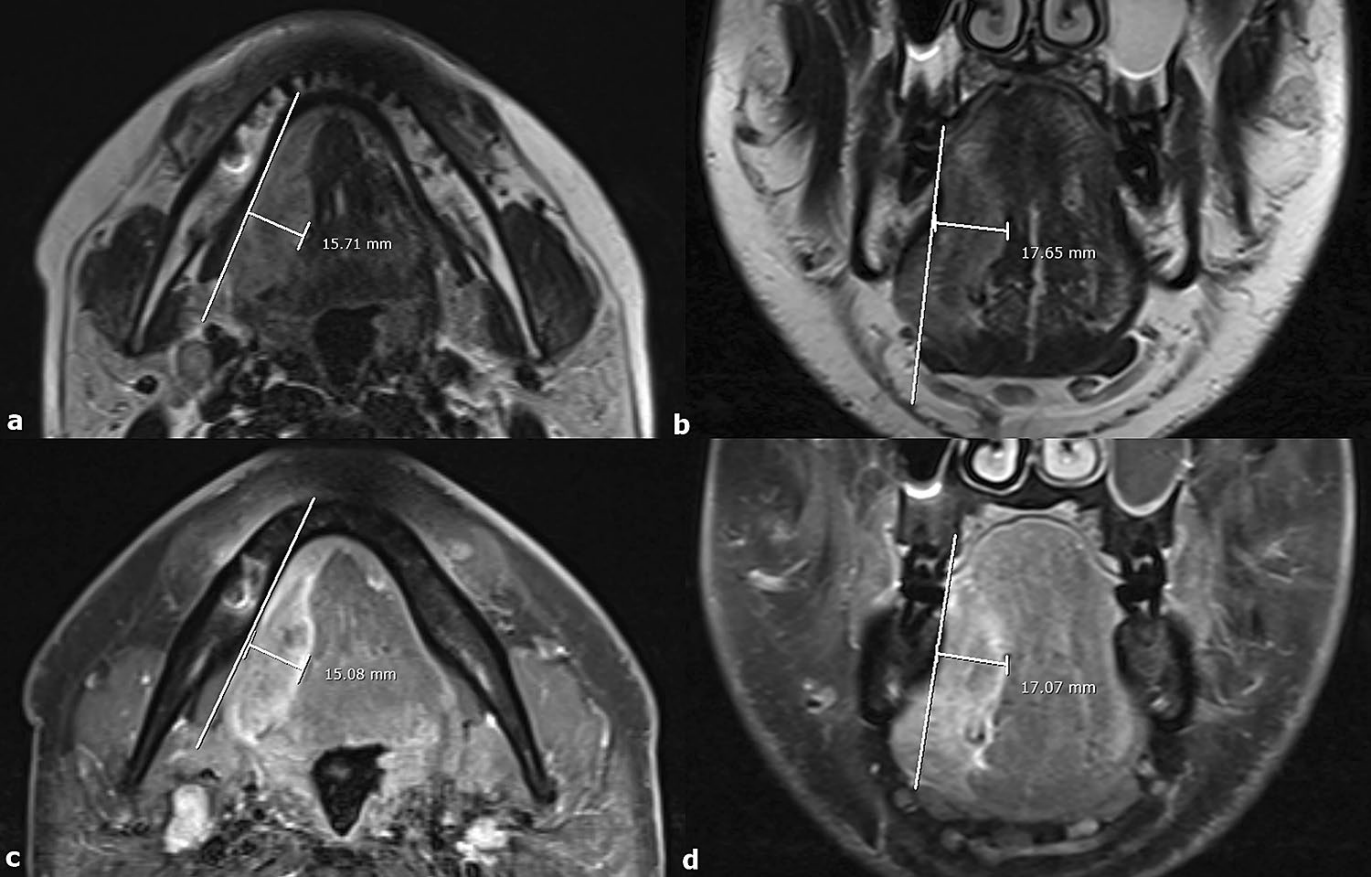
Representative measurements of MRI-measured tumor thickness (MRI-TT) on (a) axial T2WI, (b) coronal T2WI, (c) axial CE-T1WI with fat suppression, and (d) coronal CE-T1WI with fat suppression in a 39-year-old male patient diagnosed with tongue squamous cell carcinoma.
